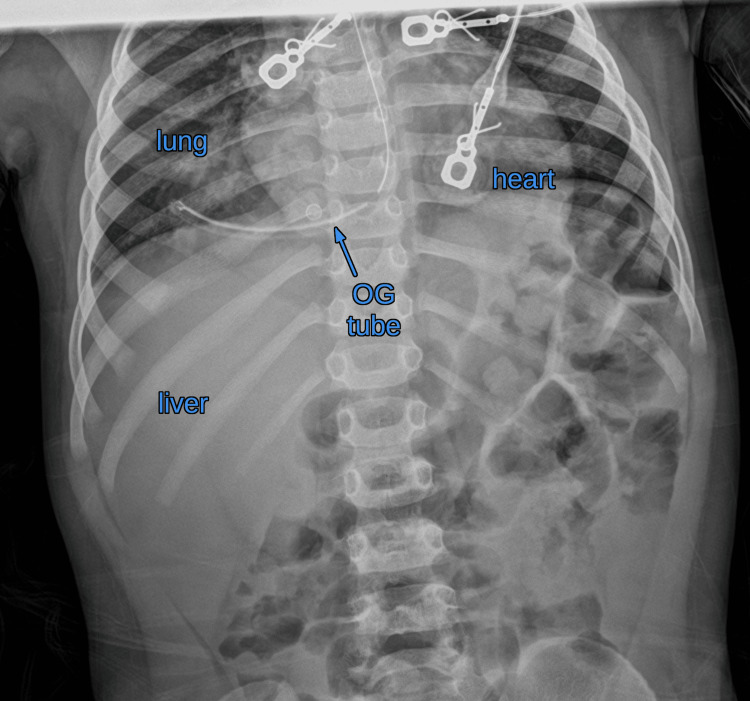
X-ray (anteroposterior view) of the lower chest and abdomen showing the orogastric (OG) tube turning towards the right side.
